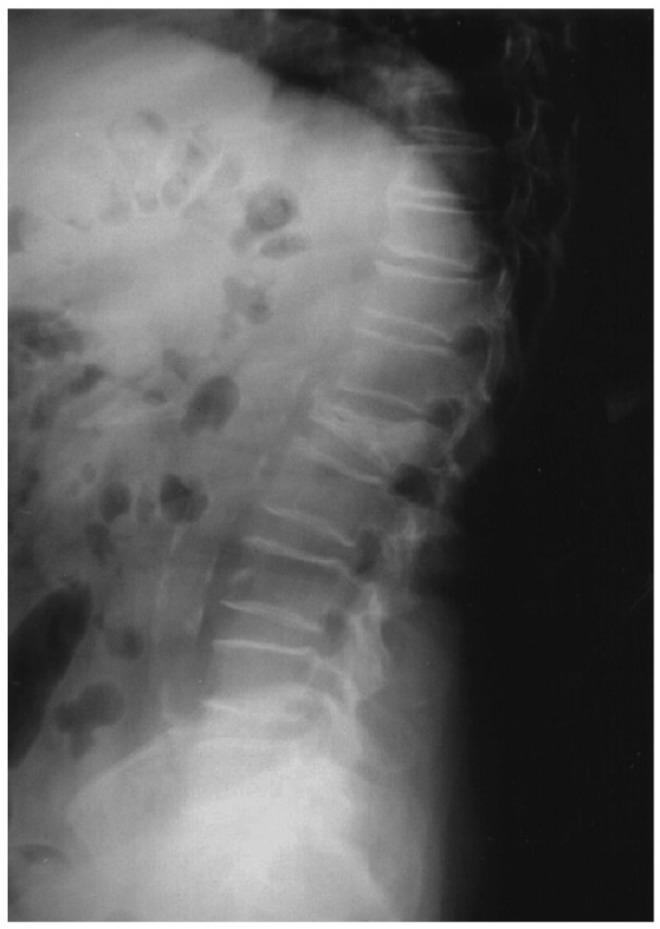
X-ray image of a 71 years old woman with aortic calcifications, low bone density and a vertebral fracture.
